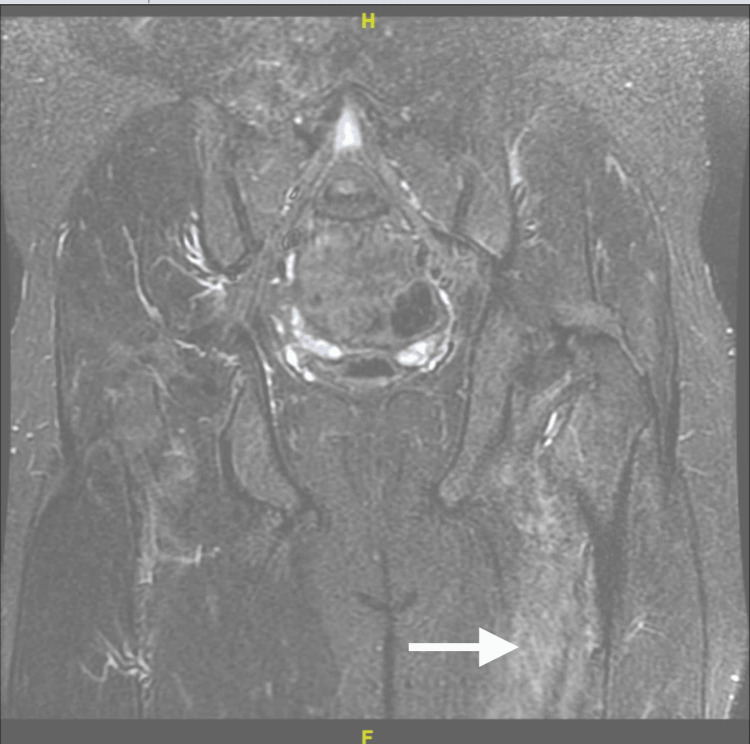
MRI of the lower extremity showing focal areas of intramuscular edema, including the lower lumbar spine posterior paraspinal musculature and bilateral pelvic musculature (left greater than right), concerning infectious myositis versus significant muscle strain.
